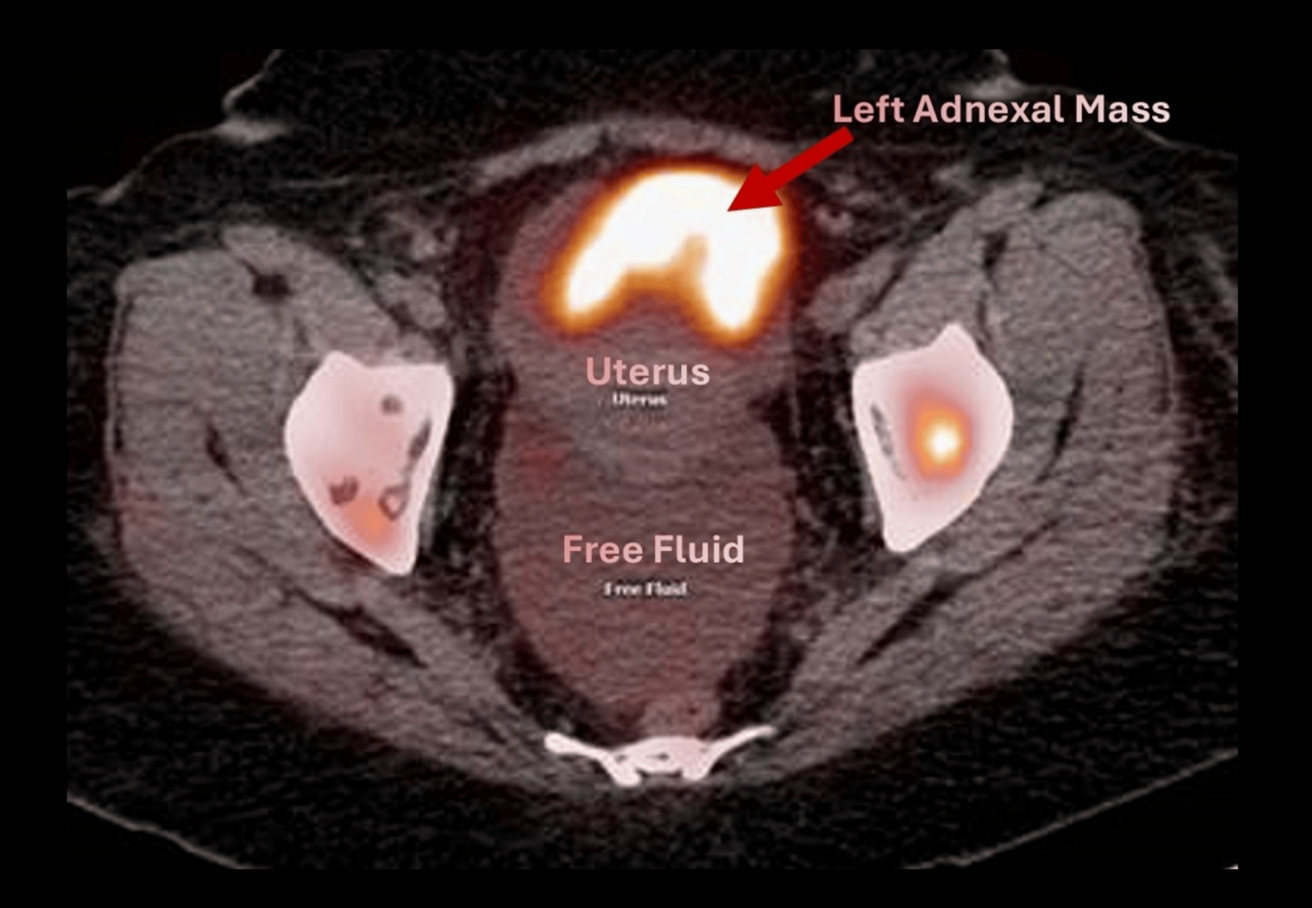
CT-PET image (axial view) CT-PET: computed tomography-positron emission tomography The overlay PET/CT scan on the right shows the left adnexal mass as well as free fluid in the pelvis in the axial view
